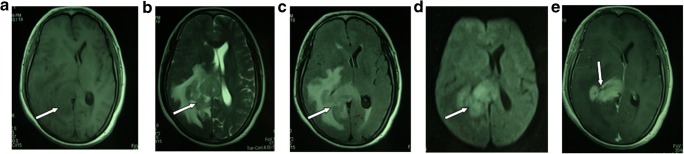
Male, 47 years of age, 1-month history of mental abnormalities. The arrow points to the typical magnetic resonance imaging (MRI) features of primary central nervous system lymphoma (PCNSL). a T1-weighted imaging. b T2-weighted. c Fluid attenuation inversion recovery (FLAIR). d Diffusion-weighted imaging (DWI). e Significant enhancement of the lesion on Gd-DTPA MRI with a typical “belly button” sign
